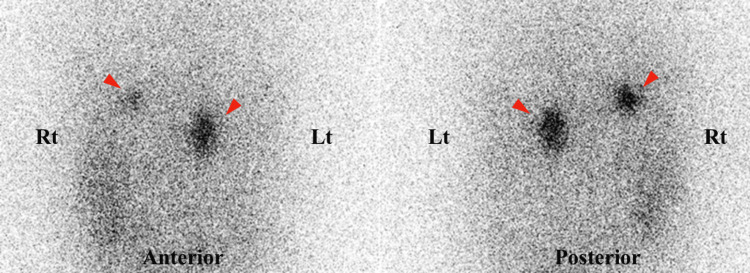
131I-Adosterol scintigraphy Accumulation in both adrenal glands (arrowheads), but exhibiting partial loss of the right adrenal gland. Rt and Lt represent right and left, respectively.
